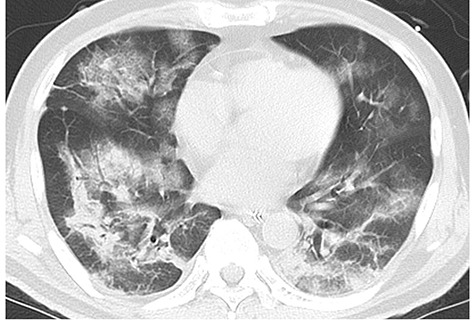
Chest CT at the onset of COVID-19 showing bilateral patchy ground-glass opacities and infiltrative shadows.
